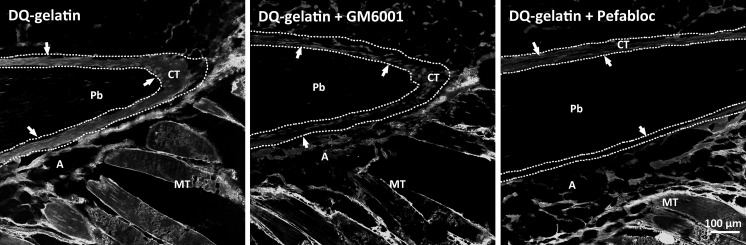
In situ zymography of salmon pin bone tissue section with DQ-gelatin in presence or absence of the universal MMP inhibitor GM6001 and the serine protease Pefabloc. *Arrows point* out the CT surrounding the pin bone. *Pb* pin bone; *CT* connective tissue; *A* adipose tissue; *MT* muscle tissue. *Scale bars* as indicated
